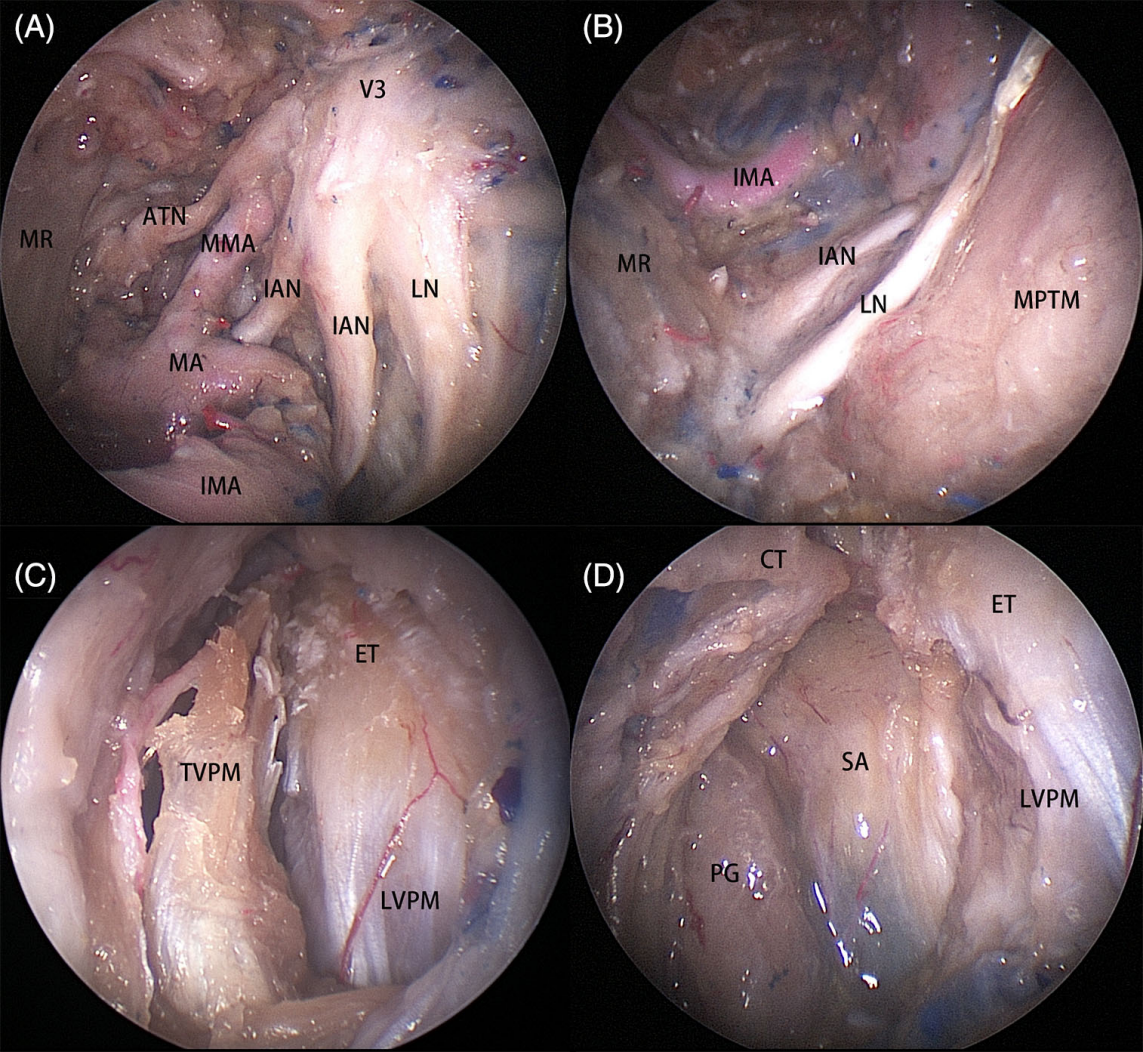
Structures on right side. (A) The branches of posterior trunk of V_3_ includes the lingual nerve (LN), inferior alveolar nerve (IAN), and auriculotemporal nerve (ATN); (B) the LN and IAN travel to the inferolateral aspect; (C) the tensor veli palatini muscle (TVPM) was incised to expose the Eustachian tube (ET) and levator veli palatini muscle (LVPM); (D) the deep lobe of parotid gland (PG) and styloid aponeurosis (SA). CT, chorda tympani; IMA, internal maxillary artery; MMA, middle meningeal artery; MPTM, medial pterygoid muscle; MR, mandible ramus [Color figure can be viewed at wileyonlinelibrary.com]
